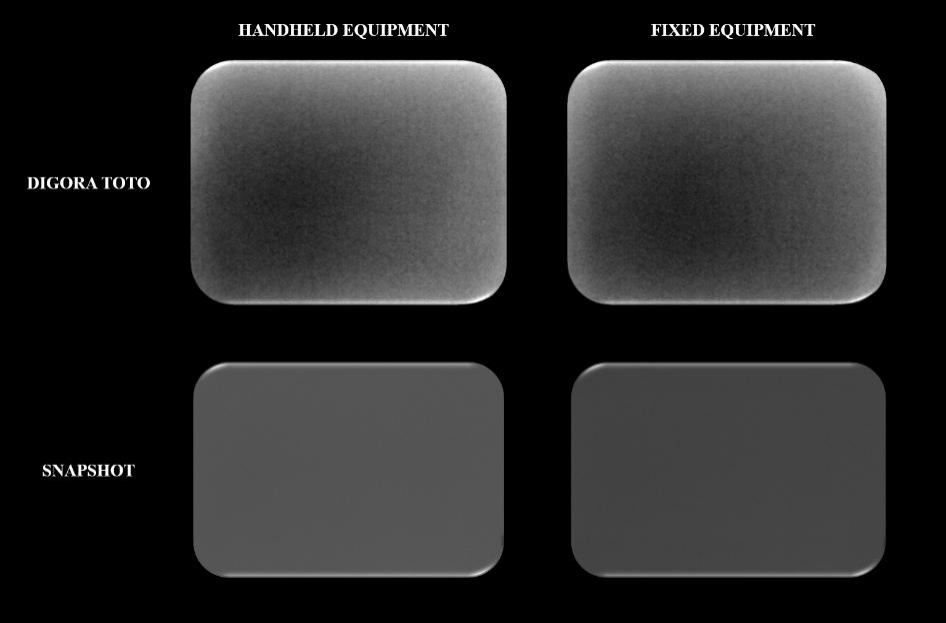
Radiographs of an acrylic block obtained with handheld portable X-ray and fixed intraoral X-ray equipment using the Digora Toto and Snapshot systems.
